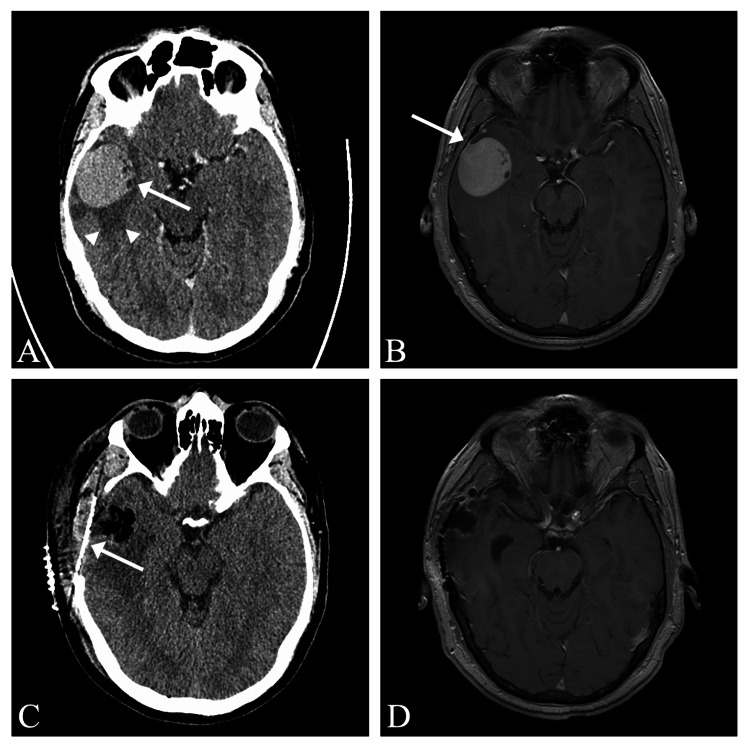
Pre- and post-operative neuroimaging A) Contrast-enhanced CT head showing a well-circumscribed right temporal lesion (white arrow) with homogenous contrast enhancement and mild associated edema (white arrowheads delineate edema); B) T1W post-gadolinium MRI showing a right temporal lesion with homogenous contrast enhancement and dural attachment (white arrow); C) Post-operative CT head showing gross total resection of the lesion with titanium mesh cranioplasty in place (white arrow); D) T1W post-gadolinium MRI three months post-operatively showing no evidence of residual or recurrent enhancing disease.
